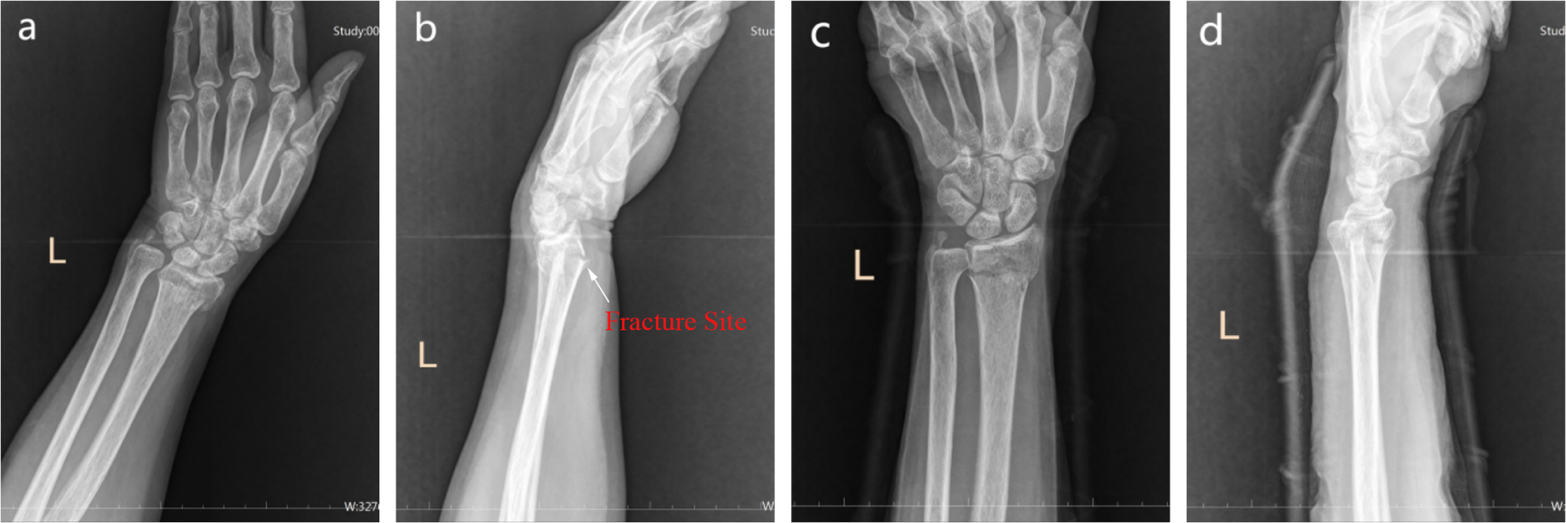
Anteroposterior and lateral wrist DRs from the emergency department and after reduction. (a,b) emergency DRs showing distal radius and ulna fractures, with radial dorsal displacement of the radius and a distal ulnar styloid fracture. (c,d) post-reduction DRs demonstrating satisfactory reduction of the radius, with evidence of DRUJ separation. DR, digital radiograph; DRUJ, distal radioulnar joint.
